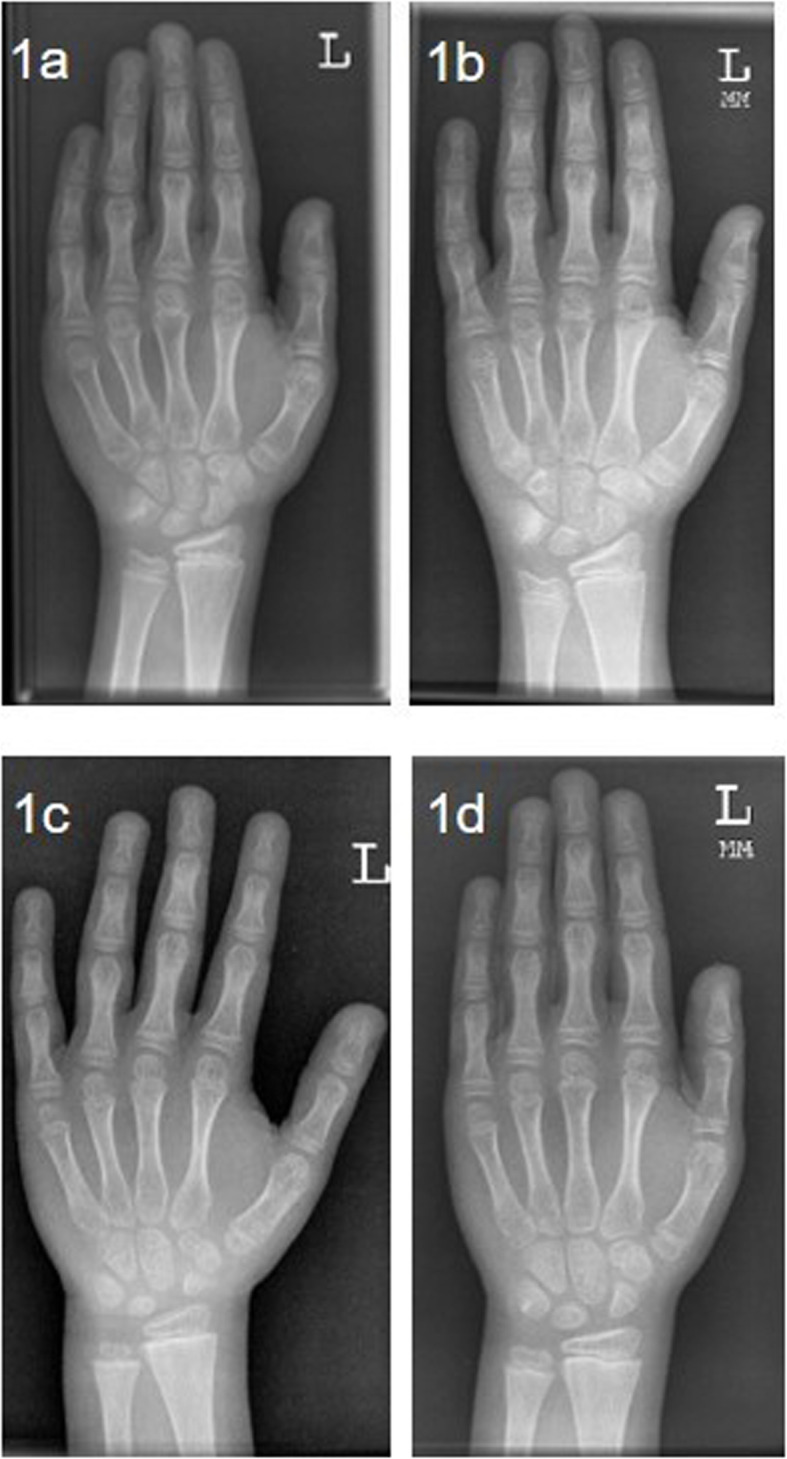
a Patient 1: Hand and wrist radiography 9y: accelerated bone development approx. 11y; b Patient 1: Hand and wrist radiography 10y 10 m: accelerated bone development approx. 12y 6 m; c Patient 2: Hand and wrist radiography 5y 9 m: slightly accelerated bone development approx. 7y; d Patient 2: Hand and wrist radiography 7y 8 m: slightly accelerated bone development approx. 9y
